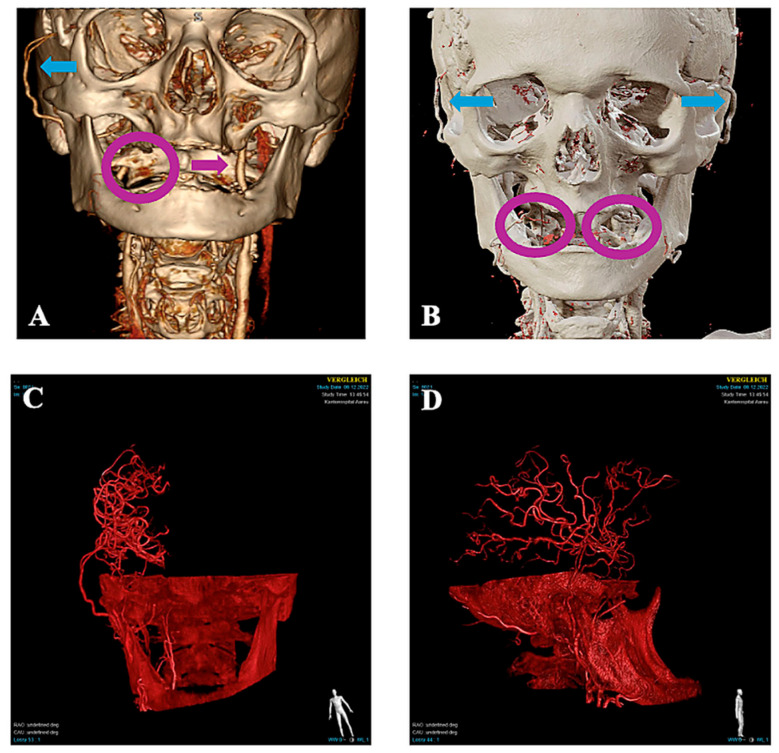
Three-dimensional postoperative CT angiography reconstructions after STA-MCA bypass surgery. (A) Reconstruction after right-sided DB STA-MCA bypass surgery. (B) Reconstruction after the second surgery, demonstrating DB STA-MCA bypasses on both sides. Blue arrows indicate the STA-MCA bypasses in (A,B). In (A), the purple circle marks the expected location of the right internal carotid artery, which is not visualized on the 3D CTA reconstruction, while the purple arrow indicates the left internal carotid artery. In (B), the purple circles mark the expected locations of both internal carotid arteries, which are not visualized on the 3D CTA reconstruction, consistent with severe steno-occlusive disease. The bottom row shows the perfusion territories of all four bypasses: (C) right DB STA-MCA bypass and (D) left DB STA-MCA bypass.
